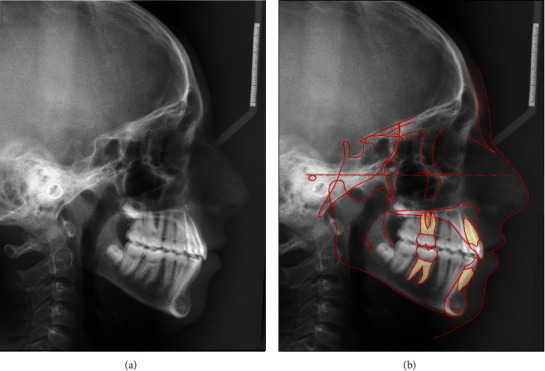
(a)Cephalometric radiograph and (b) cephalometric tracing after treatment.
